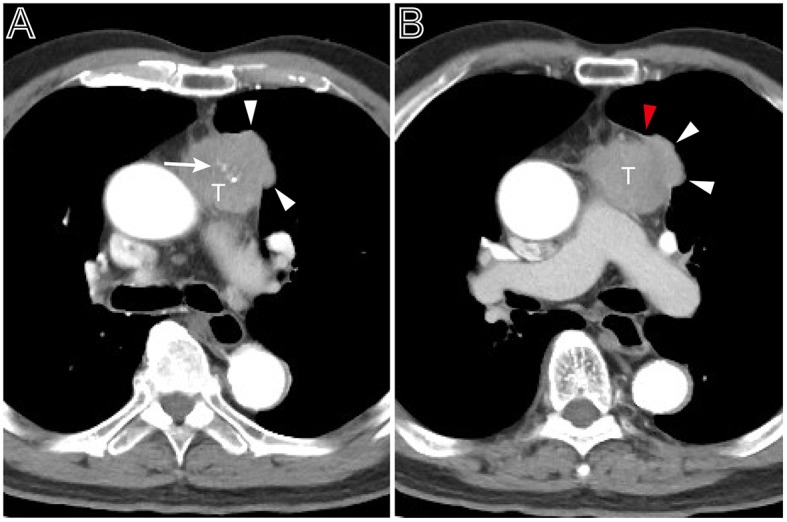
A heterogeneous thymoma accompanied with pleural nodule. (A) Axial contrast-enhanced chest CT image obtained at the level of the pulmonary trunk demonstrated a multi-lobulated thymoma with internal multiple calcification lesions (white arrow) in a 69-year-old man with myasthenia gravis (Masaoka–Koga stage IVa and WHO classification B3). (B) Showed the adjacent thickened pleura (red arrow head) and pleural nodule (white arrow head).
